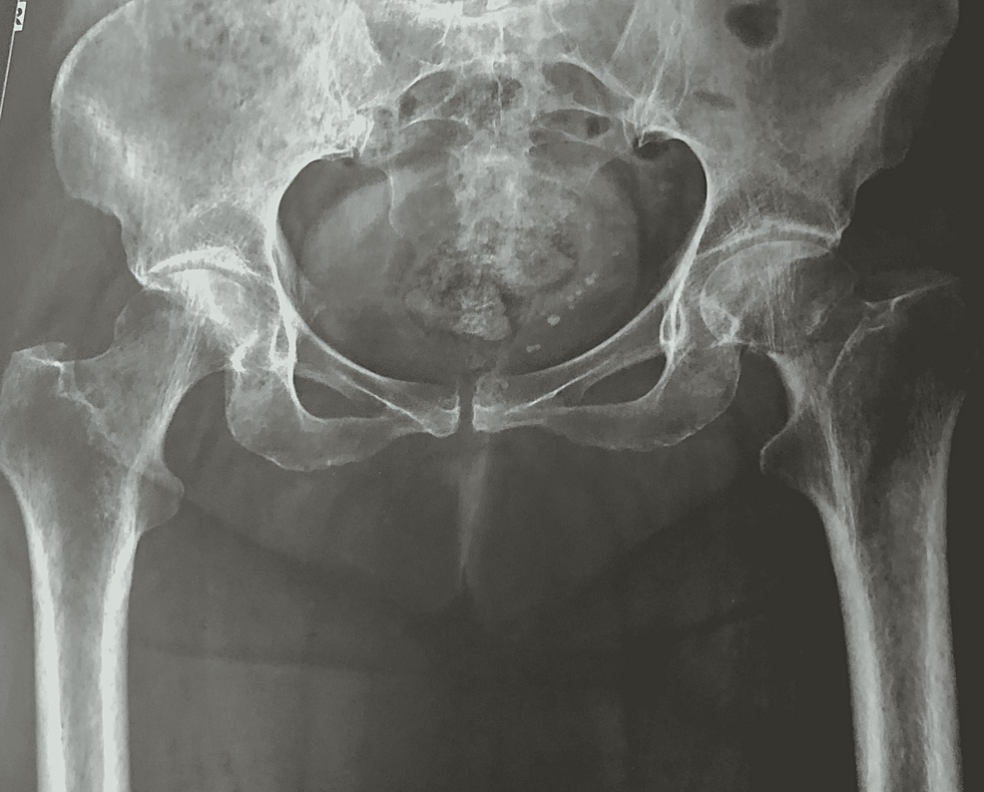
AP pelvis x-ray reveals a femoral neck fracture of the left hip
